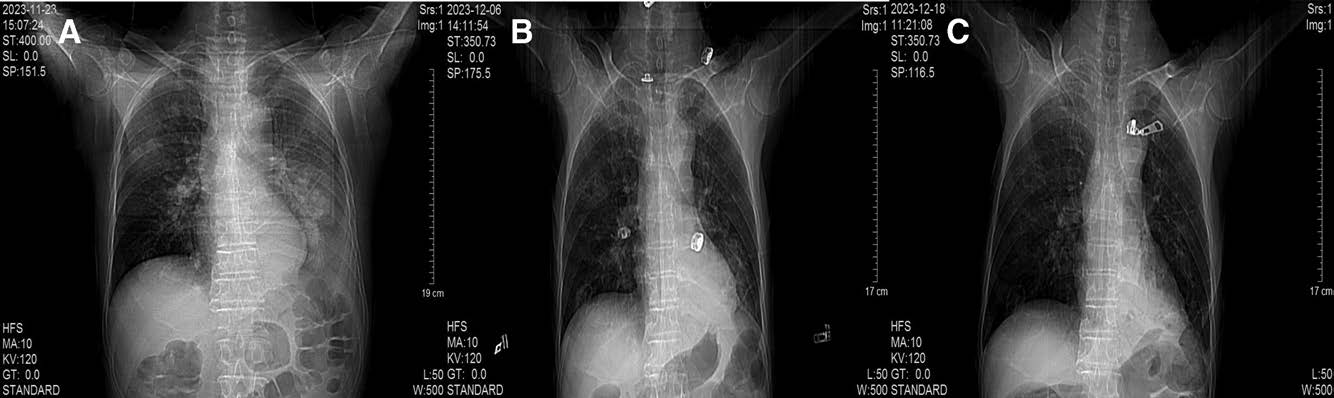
Comparison of 3 lung computed tomography results of the patient. (A) November 23rd. (B) December 6th. (C) December 18th.
